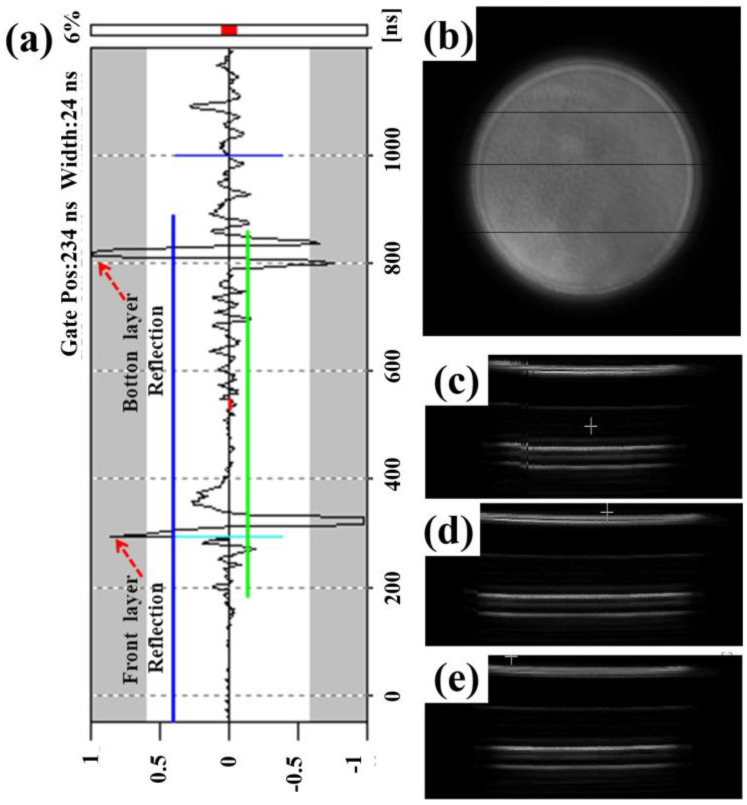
(a) Ultrasonic nondestructive reflection wave signal of the GMs; (b) internal flat structure of the GMs; (c–e) internal graded structure of the GMs.
